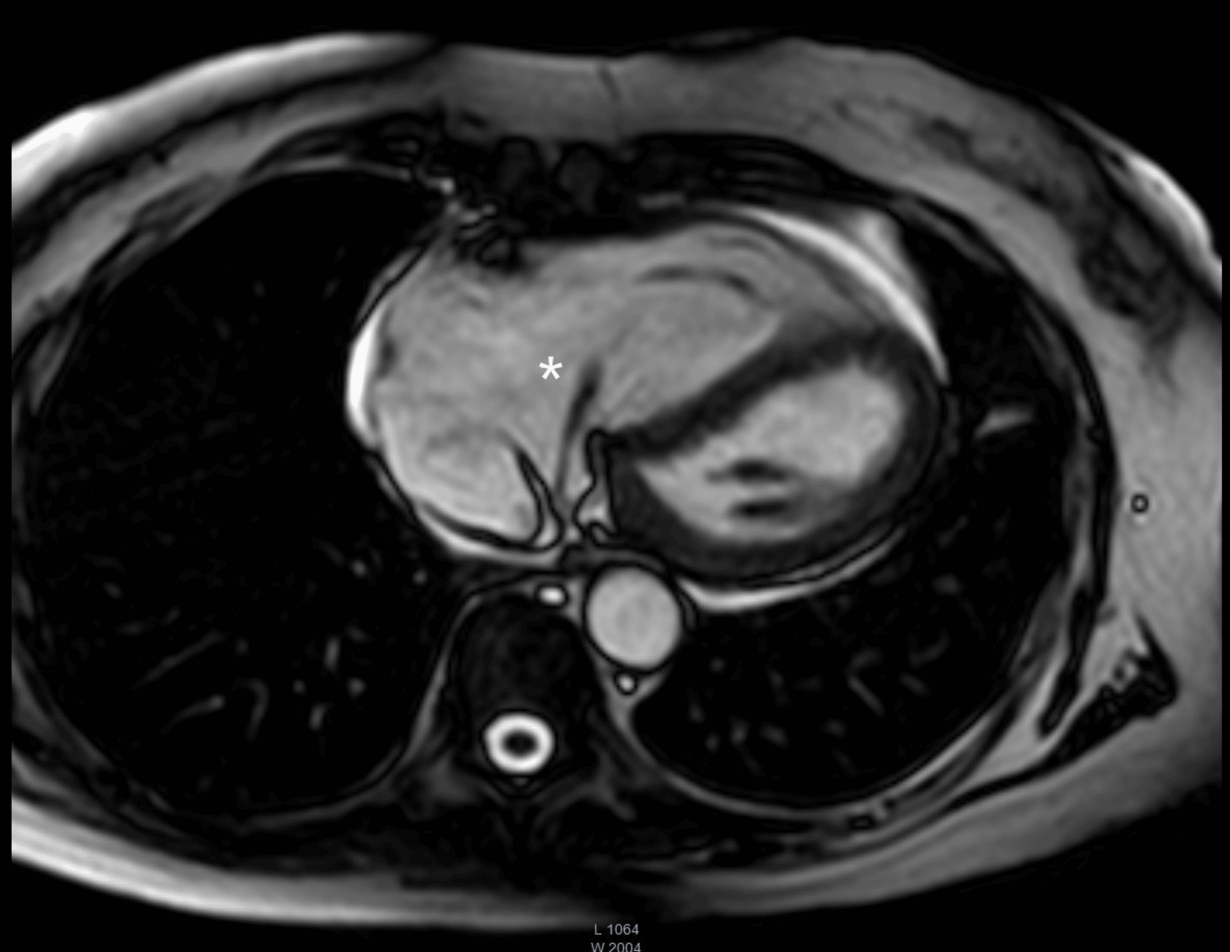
Cardiac MRI (coronal cut) showing the cardiac structure (asterisk) originating from the coronary sinus MRI: magnetic resonance imaging
